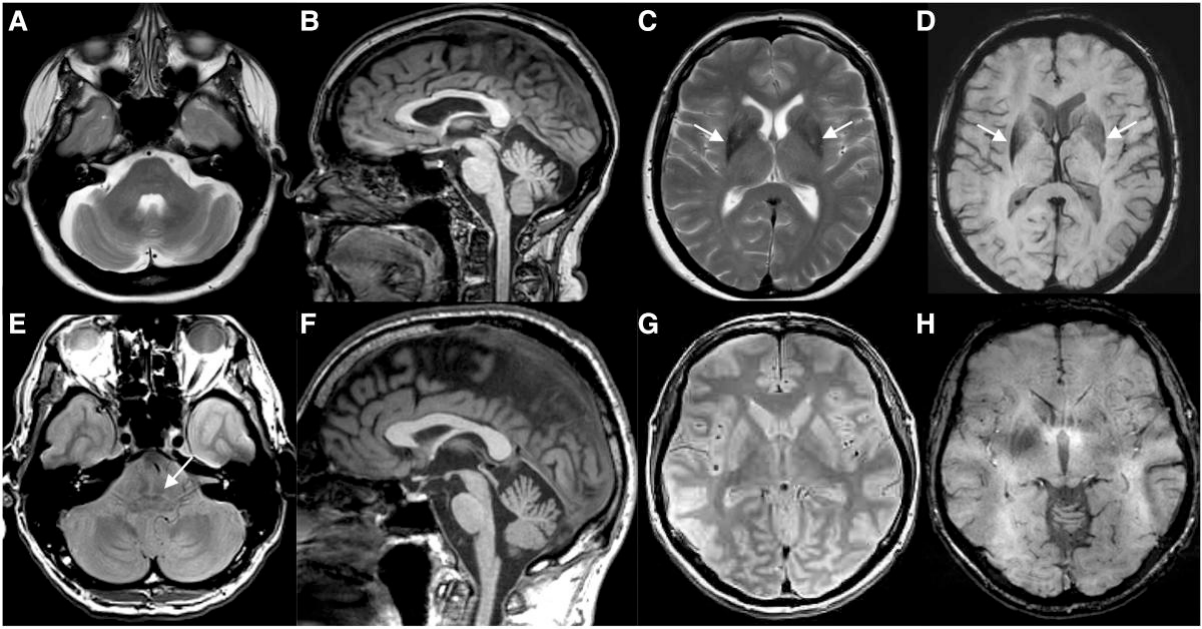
MRI features in *FGF14* GAA_≥300_ patients with MSA.  *Top panels* show MRI features in case C1 at age 59, 1 year from onset of symptoms, and *bottom panels* show MRI features in P3 at age 55, 5 years from onset of symptoms. (A and C) Axial T2-weighted images. (B and F) Sagittal T1-weighted images. (E and G) axial proton-density images. (D and H) Axial susceptibility-weighted images. Case C1 had a clinically established diagnosis of multiple system atrophy parkinsonian subtype and *FGF14* GAA_353_ repeat expansion. Case P3 had a neuropathologically established diagnosis of mixed type of multiple system atrophy and *FGF14* GAA_326_ repeat expansion. ‘Hot-cross-bun’ signs (A and E) and cerebellar atrophy (B and F) are present in both cases, more pronounced in Case P3. Hypointensity of the putamen in Case C1 is seen in is seen in C and D.
